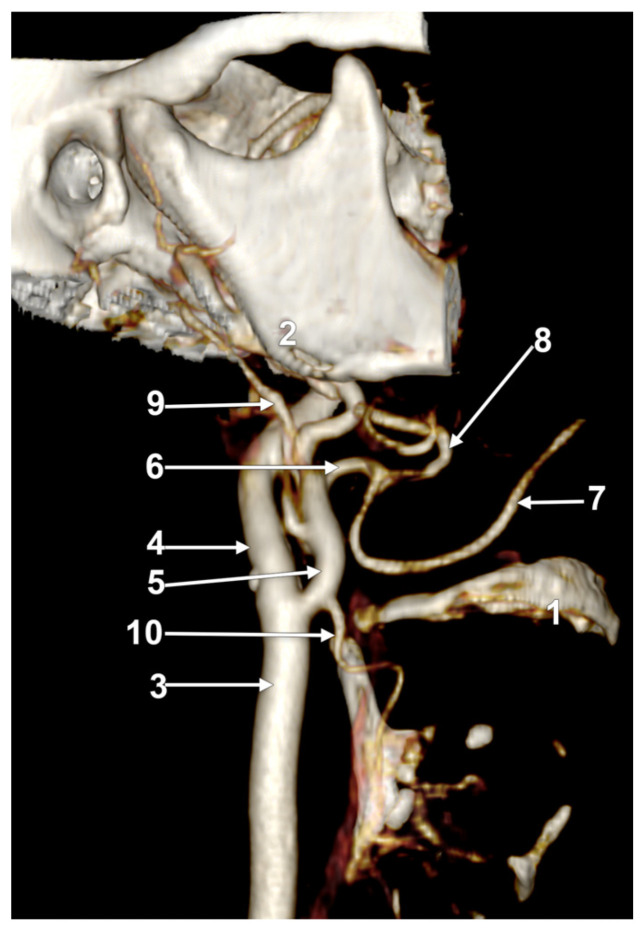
Right linguofacial trunk, CT angiogram. Three-dimensional volume rendering. Right lateral view. 1. hyoid body; 2. gonial angle; 3. common carotid artery; 4. internal carotid artery; 5. external carotid artery; 6. linguofacial trunk; 7. lingual artery; 8. facial artery; 9. occipital arteryș and 10. superior thyroid artery.
